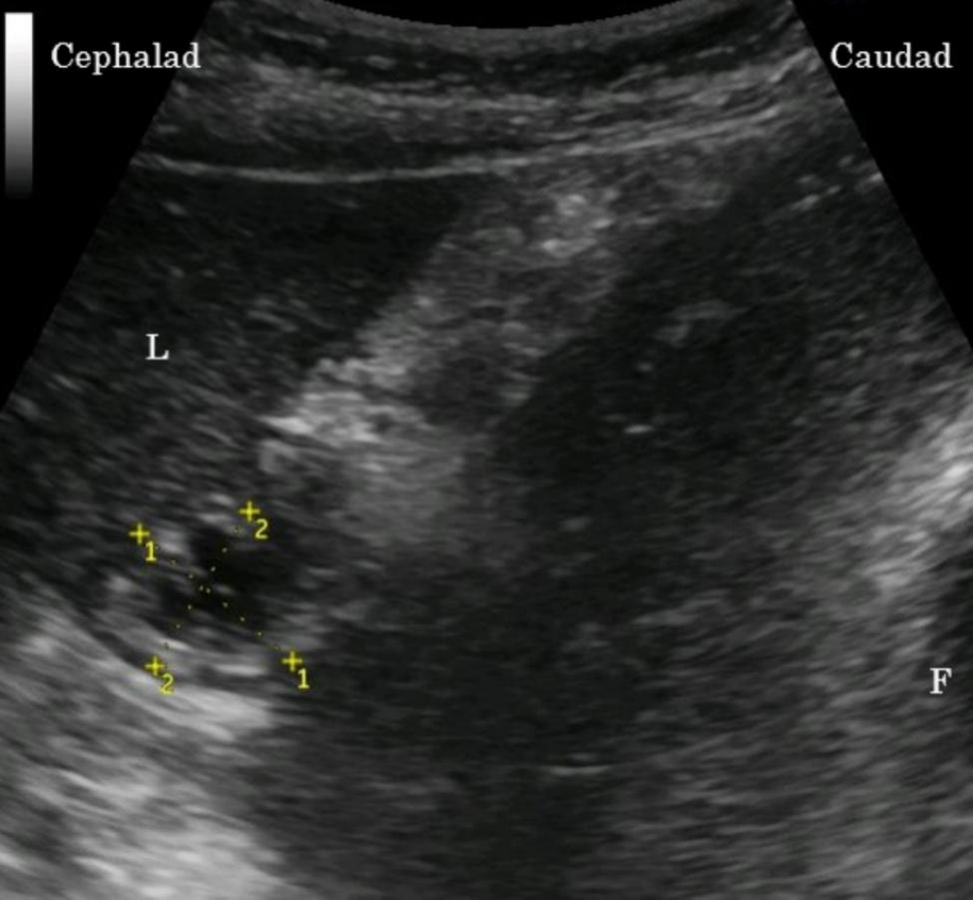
Ultrasonographic image of the gastric antrum The figure demonstrates two perpendicular diameters, line 1 and line 2, for cross-sectional area of the stomach antrum Line 1: Craniocaudal antral diameter Line 2: Anteroposterior antral diameter L, liver; F, fetal head
